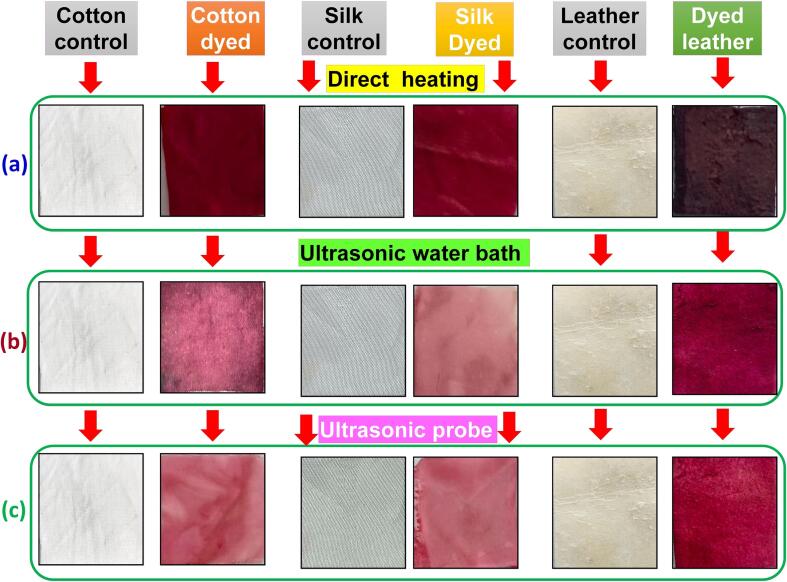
Dyeing of cotton, silk and leather using three different techniques (a) direct heating, (b) ultrasonic water bath and (c) ultrasonic probe with control samples.
